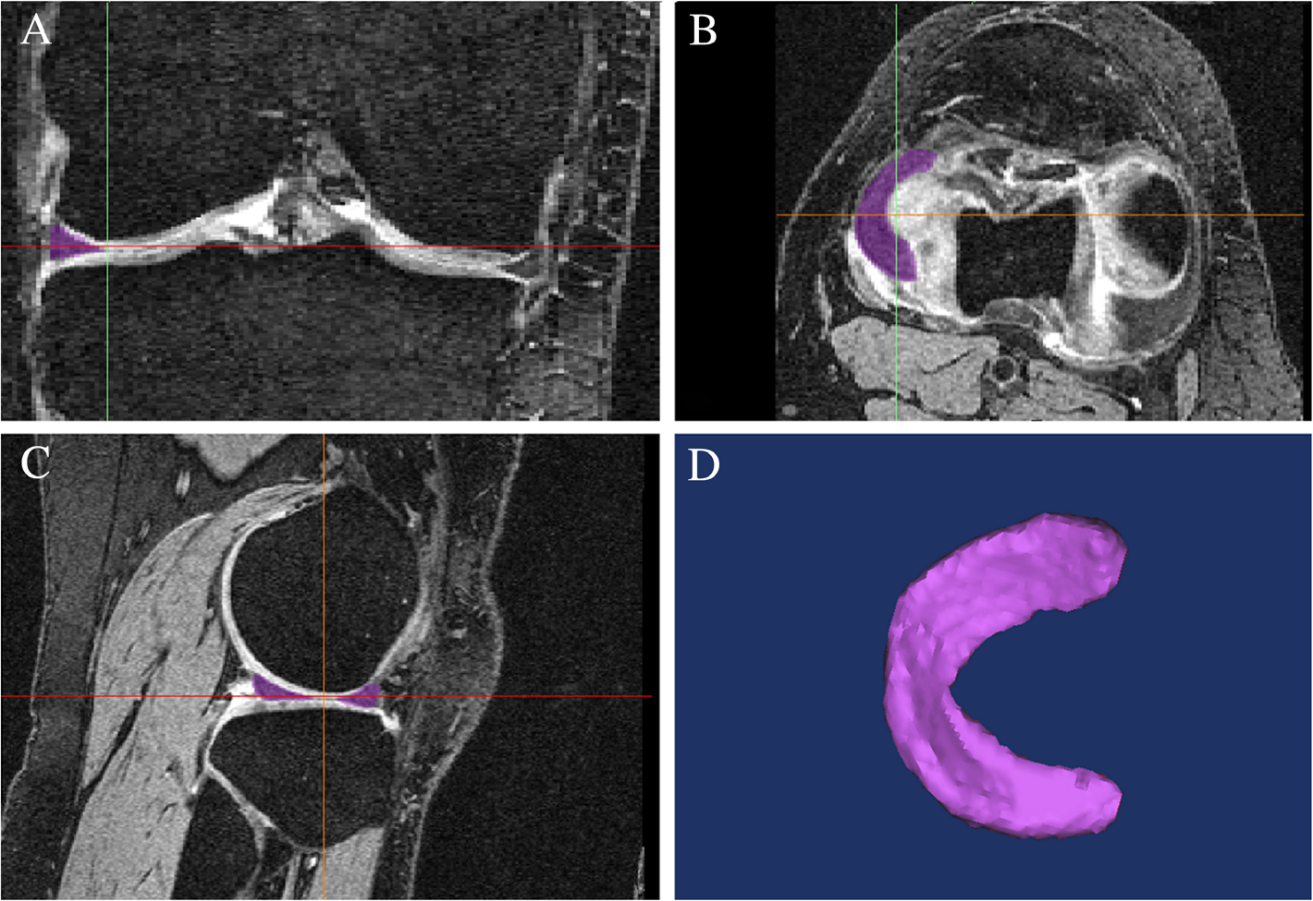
Segmentation of the magnetic resonance MR images. (A) Coronal plane;
